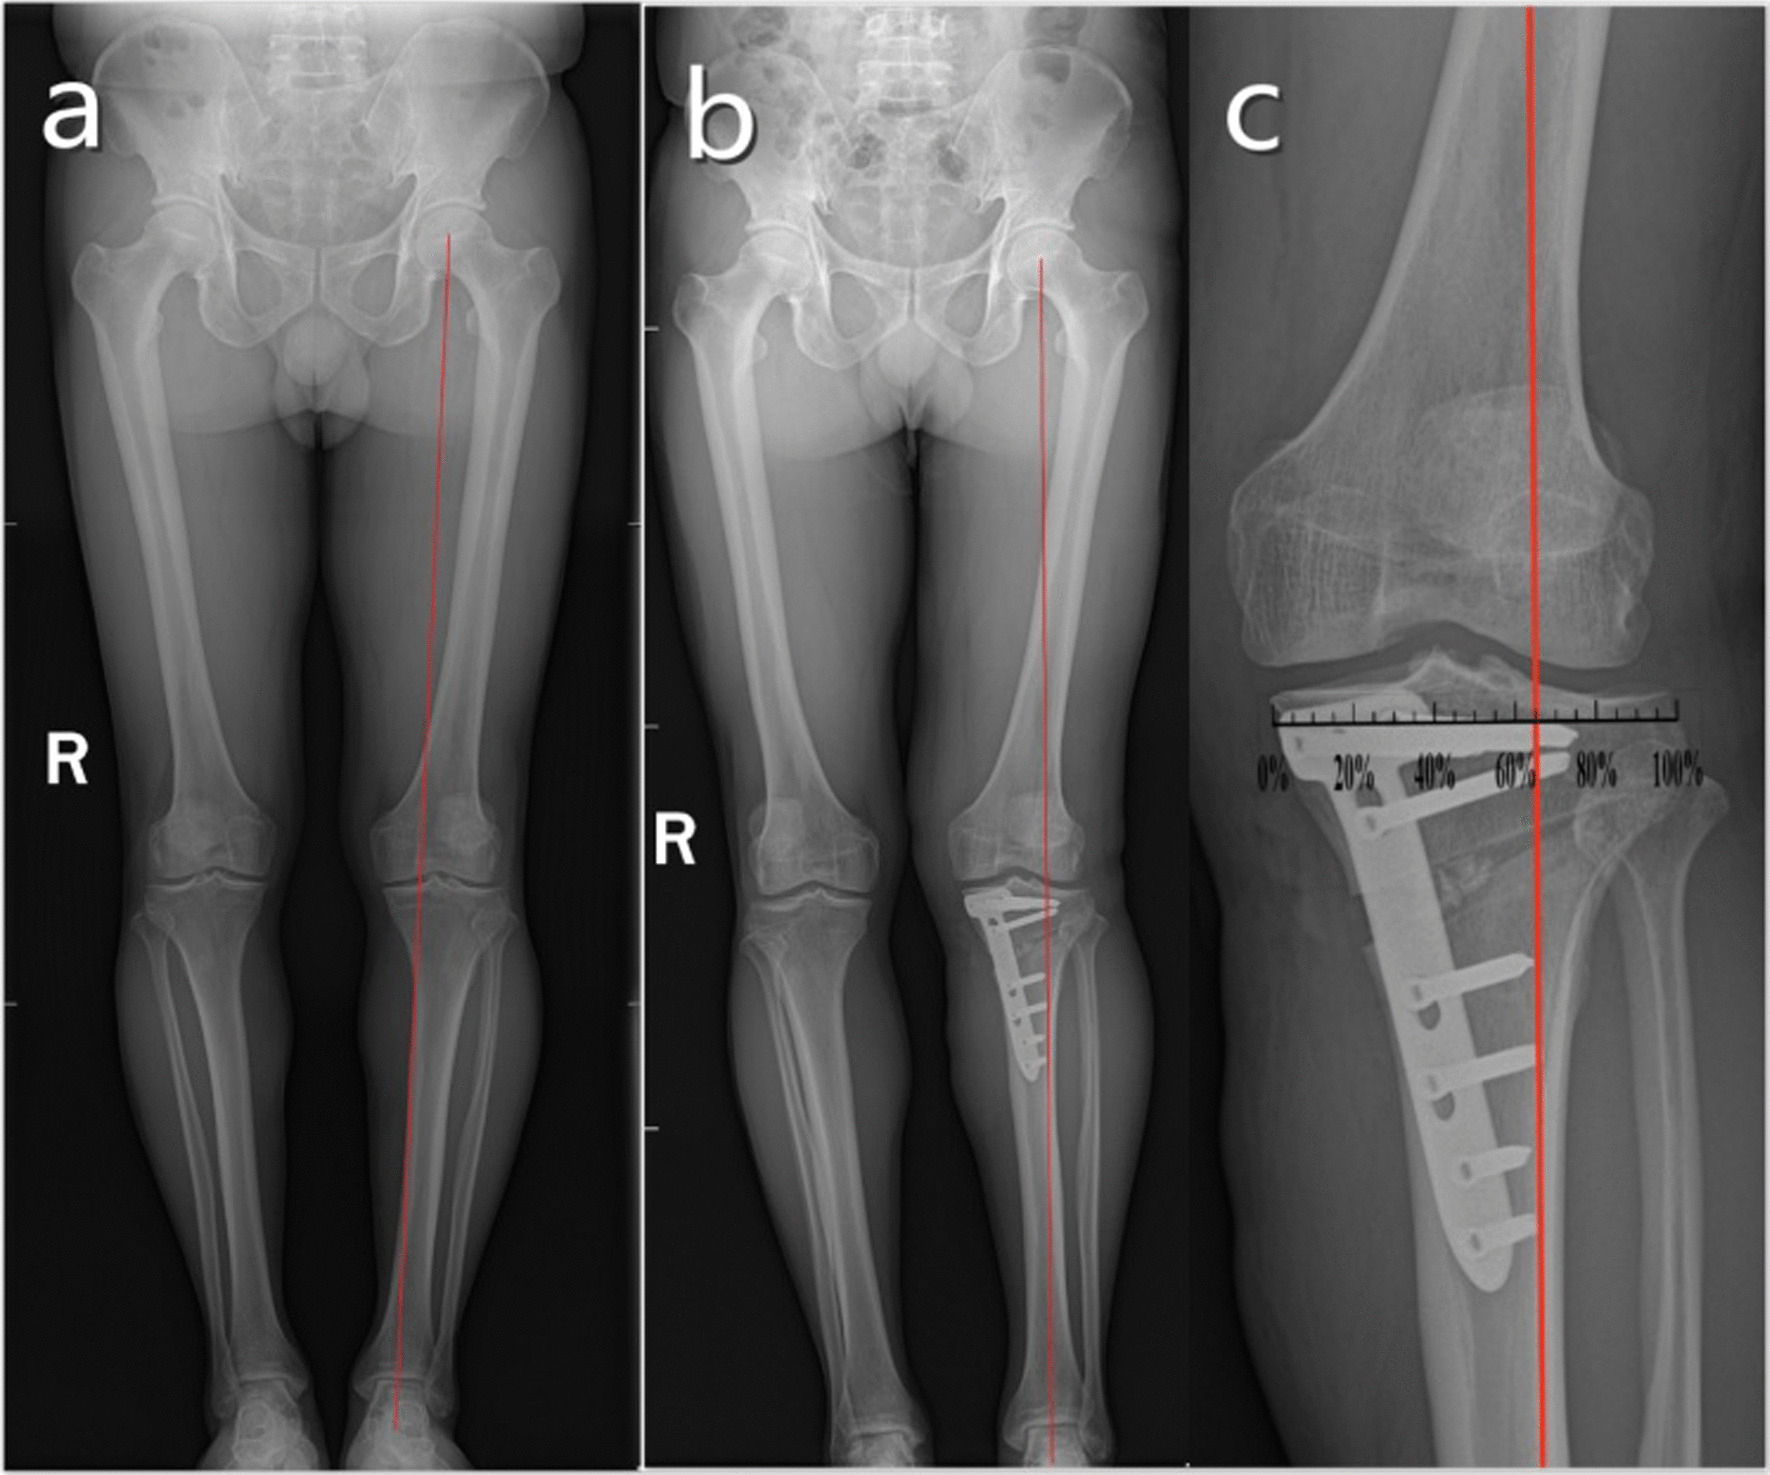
A 54-year-old male was admitted to the hospital for pain in his left knee joint for 8 months and diagnosed with knee osteoarthritis (Kellgren–Lawrence grade 3). Preoperative full-length X-rays of both lower limbs showed that the WBA of the left lower limb deviated from the medial tibial plateau (a). Postoperative X-ray of HTO showed that the WBA the left lower limb deviated to the lateral tibial plateau (b). After correction, the WBA was located at ~ 62%–66% of the tibial plateau (c)
